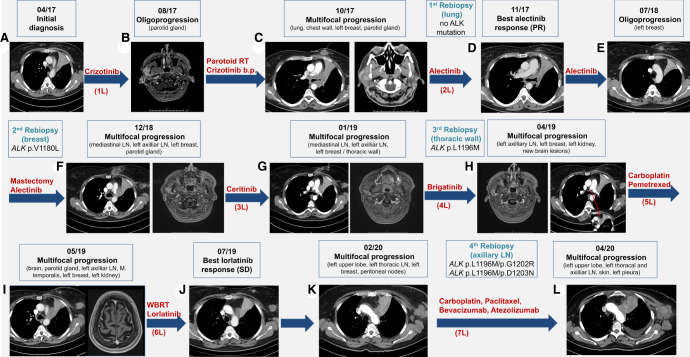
Radiologic findings over the course of the disease. (*A*) Chest computed tomography (CT) scan at initial diagnosis. (*B*) Head magnetic resonance imaging (MRI) 4 mo after crizotinib start with oligoprogression in the right parotid gland. (*C*) Chest and cranial CT scans with multifocal progression after radiotherapy. (*D*) Chest CT scan showing response to alectinib. (*E*) Chest CT scan showing oligoprogression in the left breast 8 mo later. (*F*) Chest CT and head MRI showing multifocal progression after partial mastectomy. (*G*,*H*) Chest CT and head MRI showing multifocal progression under certinib and brigatinib. (*I*) Chest CT and head MRI showing multifocal progression under carboplatin-pemetrexed. (*J*) Chest CT showing response to lorlatinib. (*K*) Chest CT and head MRI showing multifocal progression under lorlatinib. (*L*) Chest CT showing multifocal progression under carboplatin-paclitaxel-bevacizumab-atezolizumab; the patient died 3 wk later. (L) Treatment line, (RT) radiotherapy, (PR) partial response, (SD) stable disease, (LN) lymph nodes.
